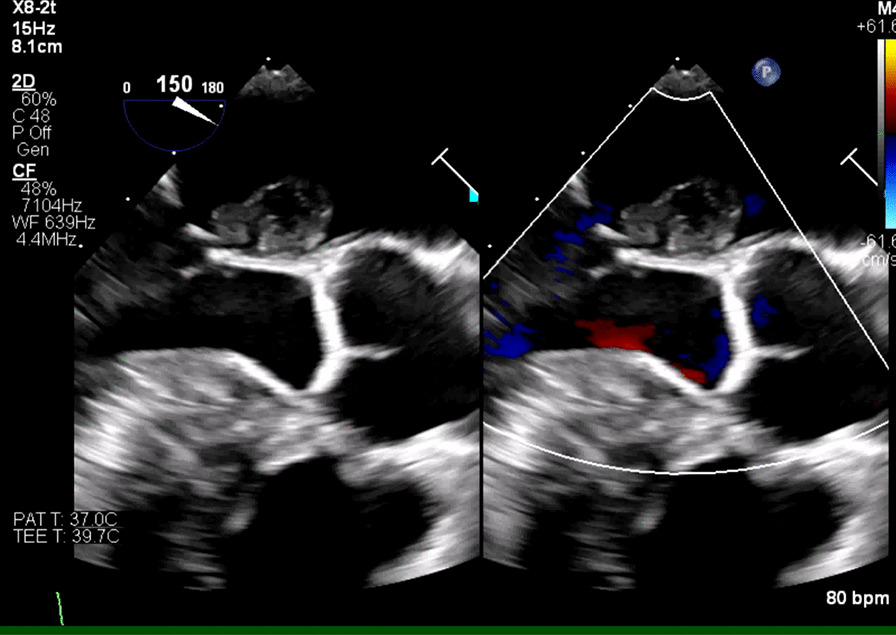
Transesophageal echocardiogram showing the polypoid echodensity on the atrial side of the Anterior mitral valve leaflet/along the aortomitral cushion
